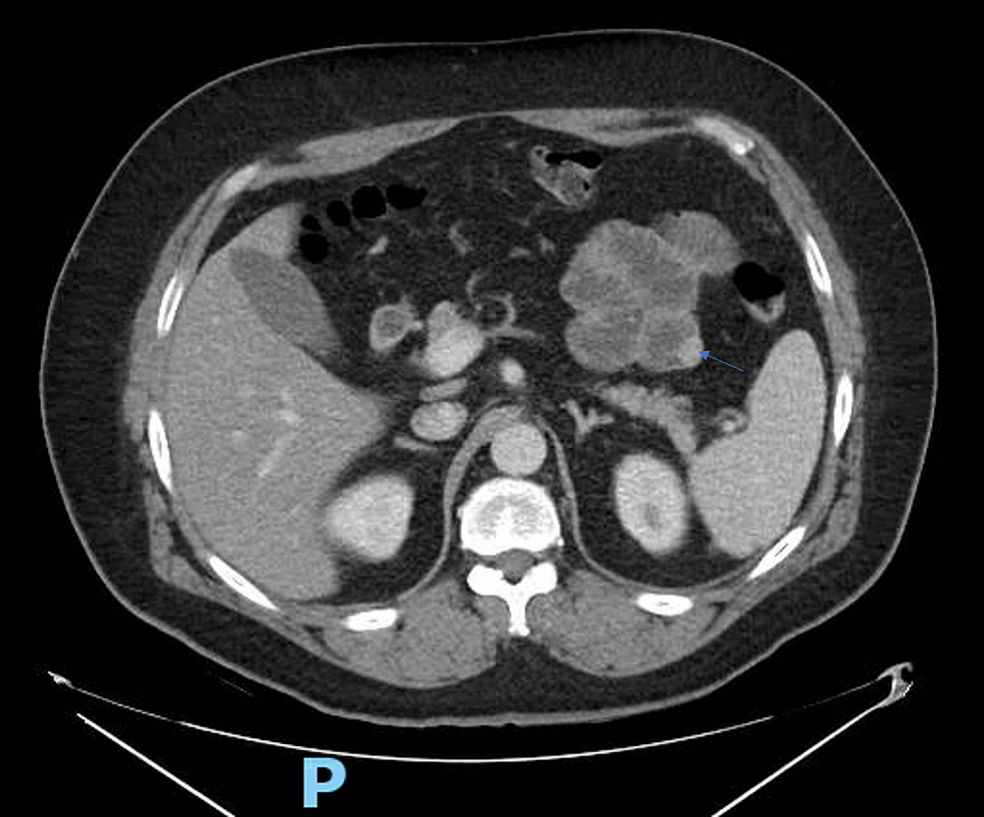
Axial section of CT abdomen with IV contrast showing 1.5 cm hyperdense, circular jejunal lesion with regular borders.
